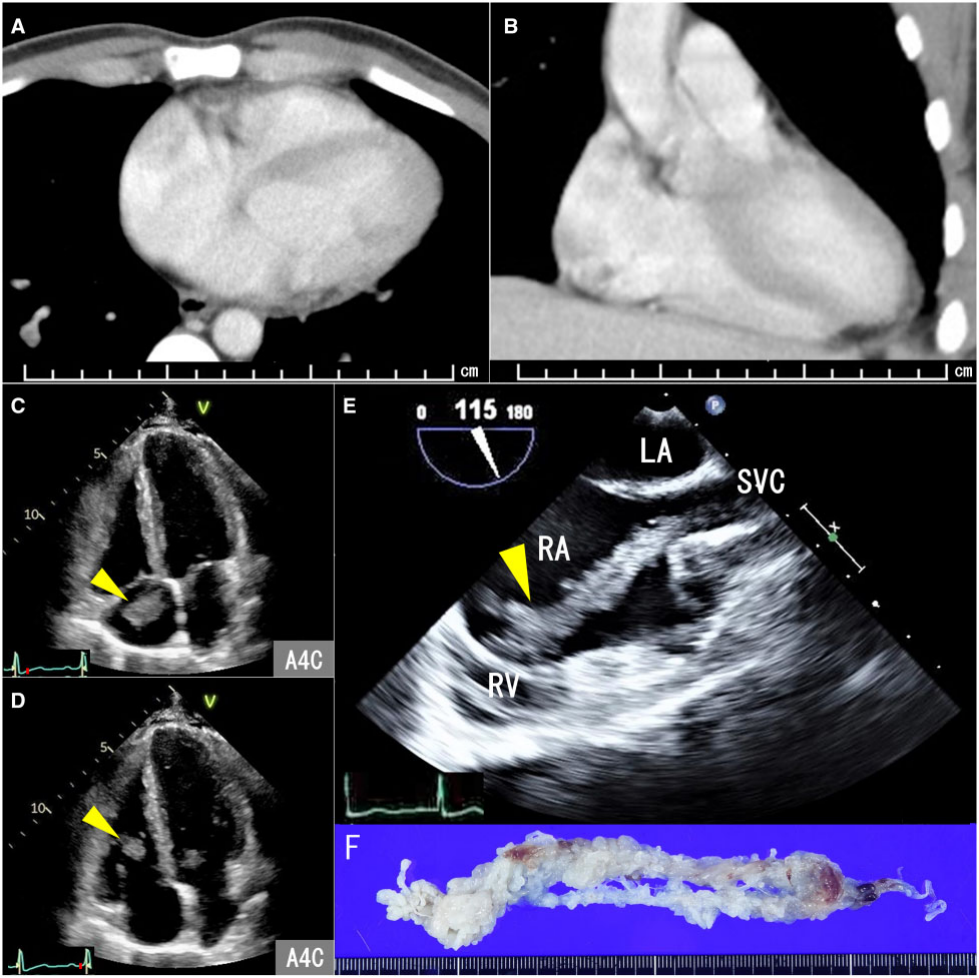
Cardiac metastases (CM) are not rare; they are encountered in 1.5–20% of autopsies of cancer patients. However, CM from testicular tumours is very rare. Computed tomography is useful for identifying CM and provides information about intra-, peri-, and extracardiac lesions. In this case, the tumour moved freely and rapidly within the cardiac cavity. Identification of such metastatic masses may be difficult on routine CT imaging. The CM was visualized on TTE, and its detailed morphology was observed on TOE. Transthoracic echocardiography and TOE are indispensable modalities to complement CT in the detection of CM, especially in an emergency case.
